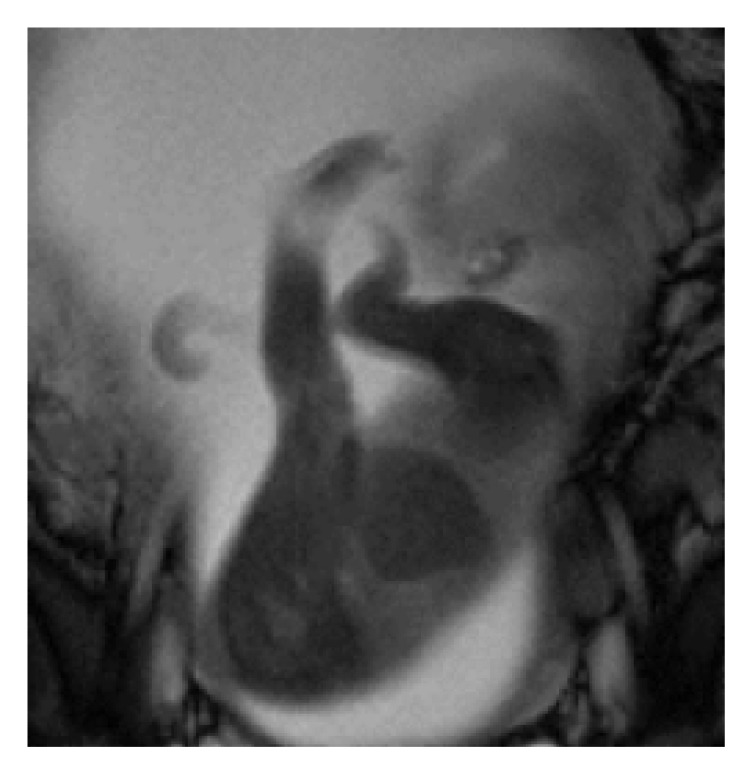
Fetal MRI at 26 weeks of gestation showing short forearms and hypoplasty of hand fingers.
